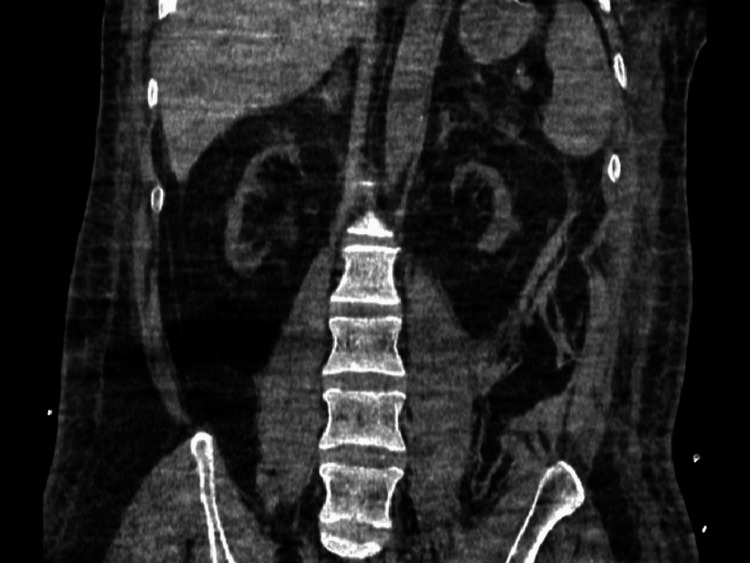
CT abdomen pelvis (coronal images) showing patient’s native end-stage kidneys.
